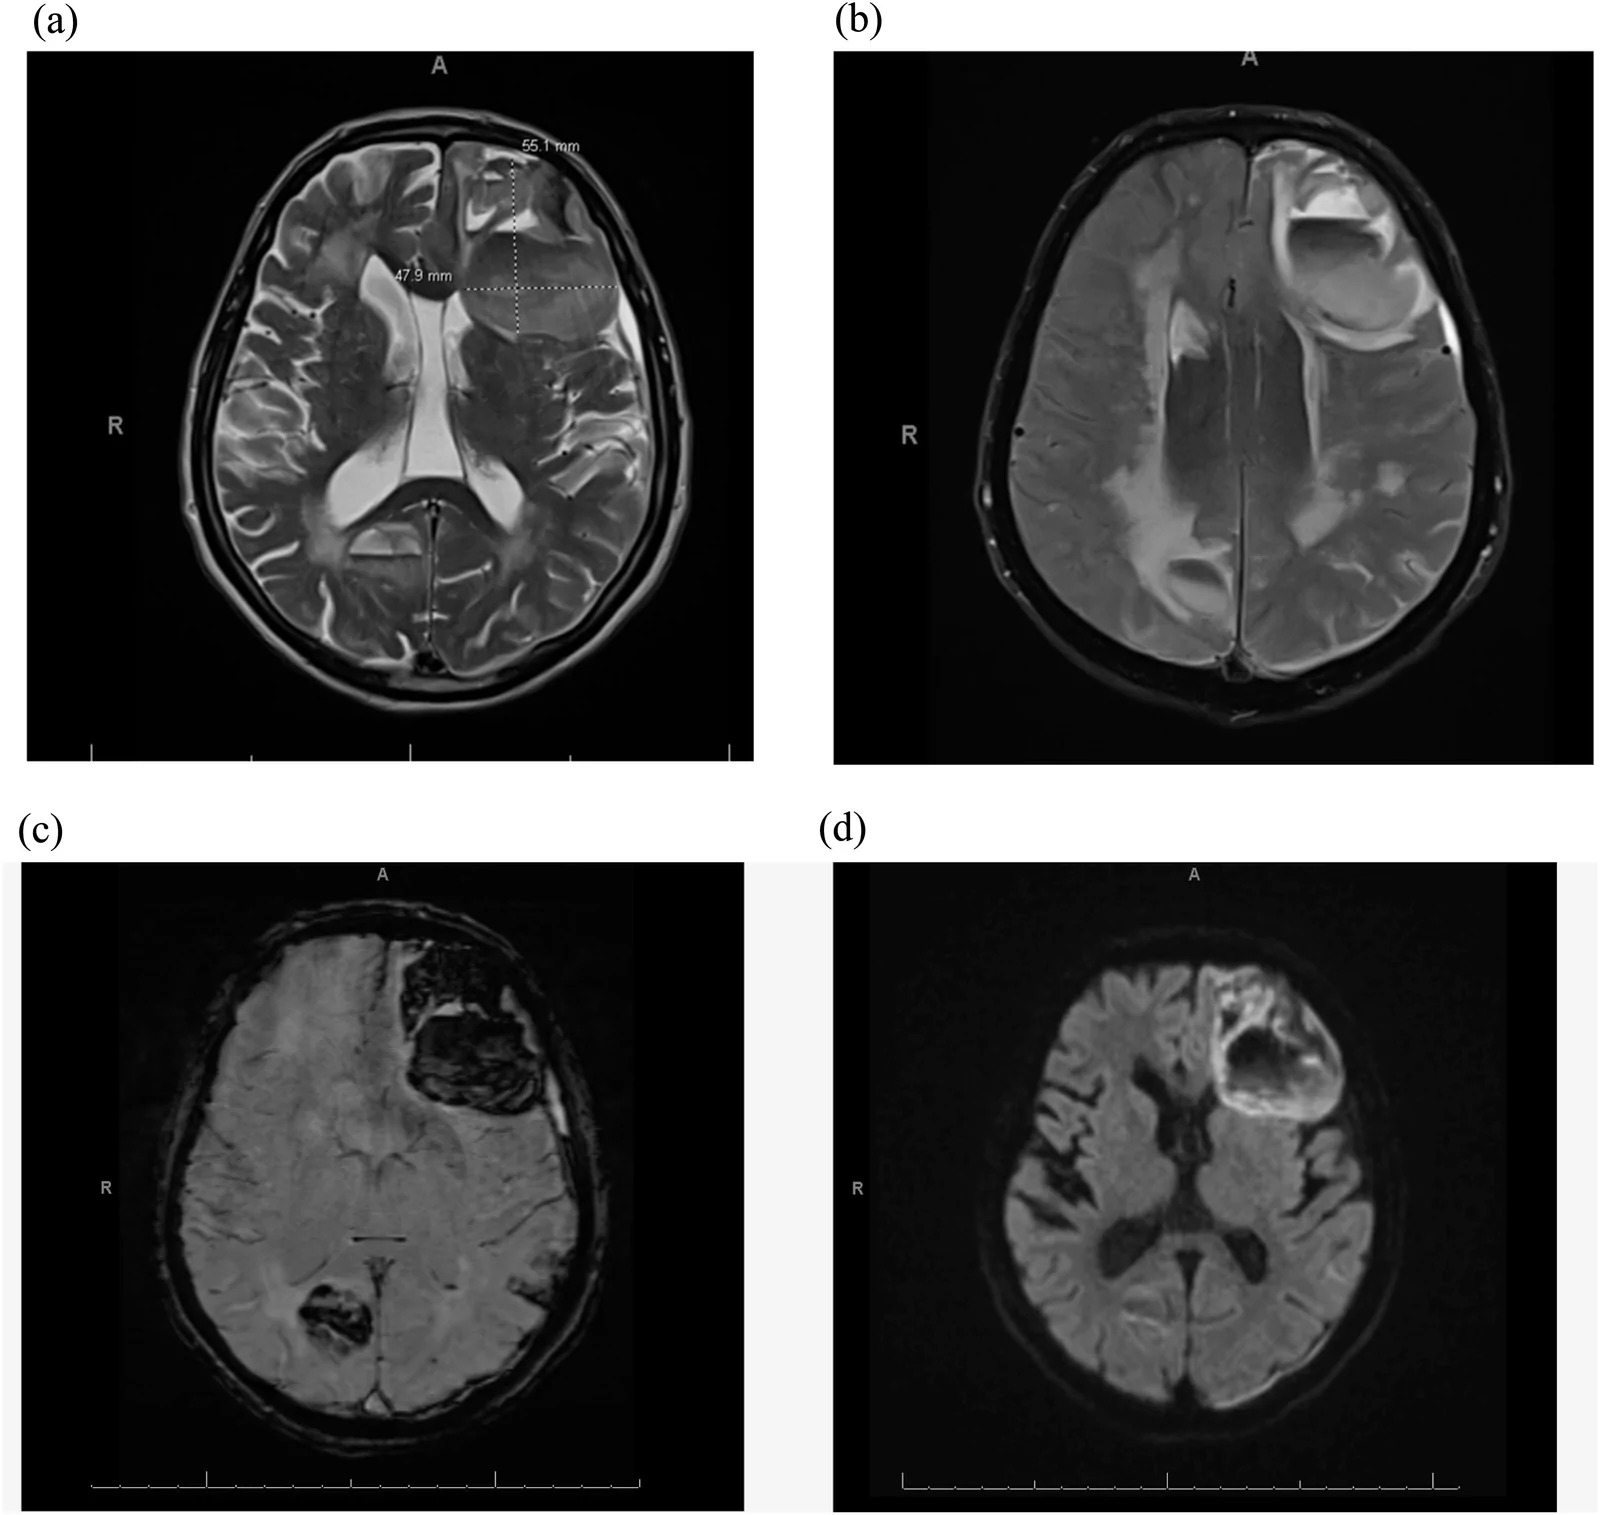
MRI findings on 11/22/2025 after tenecteplase administration. (A) Axial T2-weighted MRI demonstrated a large left frontal intraparenchymal hematoma with surrounding vasogenic edema and mass effect. (B) Axial T2/FLAIR MRI demonstrated the left frontal hematoma with adjacent vasogenic edema, additional white matter hyperintensity, and associated subarachnoid and intraventricular blood products. (C) Axial susceptibility-weighted MRI demonstrated susceptibility effects corresponding to multifocal intraparenchymal, subarachnoid, and intraventricular blood products. (D) Diffusion-weighted MRI demonstrated small cortical/juxtacortical foci of restricted diffusion in the high left frontal lobe, compatible with small acute to early subacute infarctions
